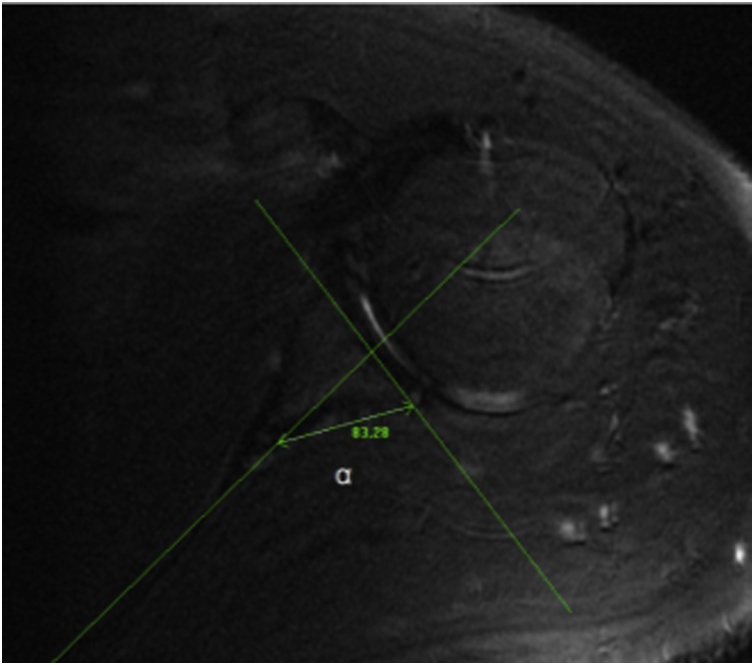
Measurement of GVA on axial MRI.
